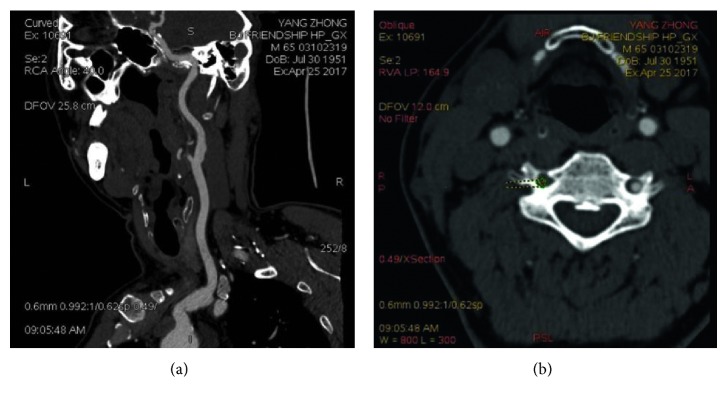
Head-and-neck CTA inspection. (a) Wall calcification of the ICAS; (b) the stenosis of the ICA.
